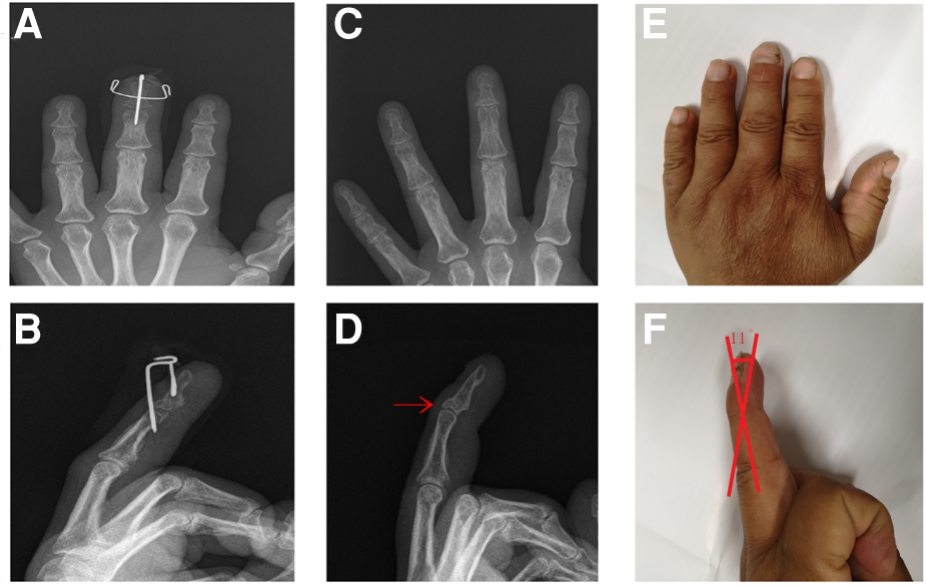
Follow-up of patient No.7. (A,B). X-ray at 8 weeks postoperatively. (C,D). X-ray at 24 weeks postoperatively;malunion (red arrow). (E,F). Finger appearance at 24 weeks; extension deficit (red angle).
